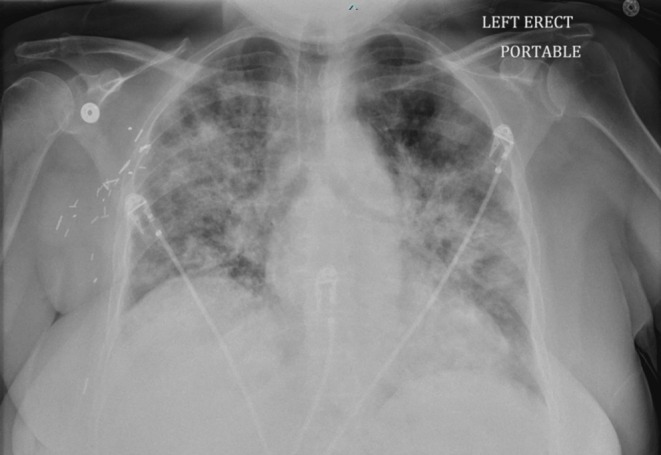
Initial chest radiograph showing diffuse interstitial and alveolar opacities bilaterally.
